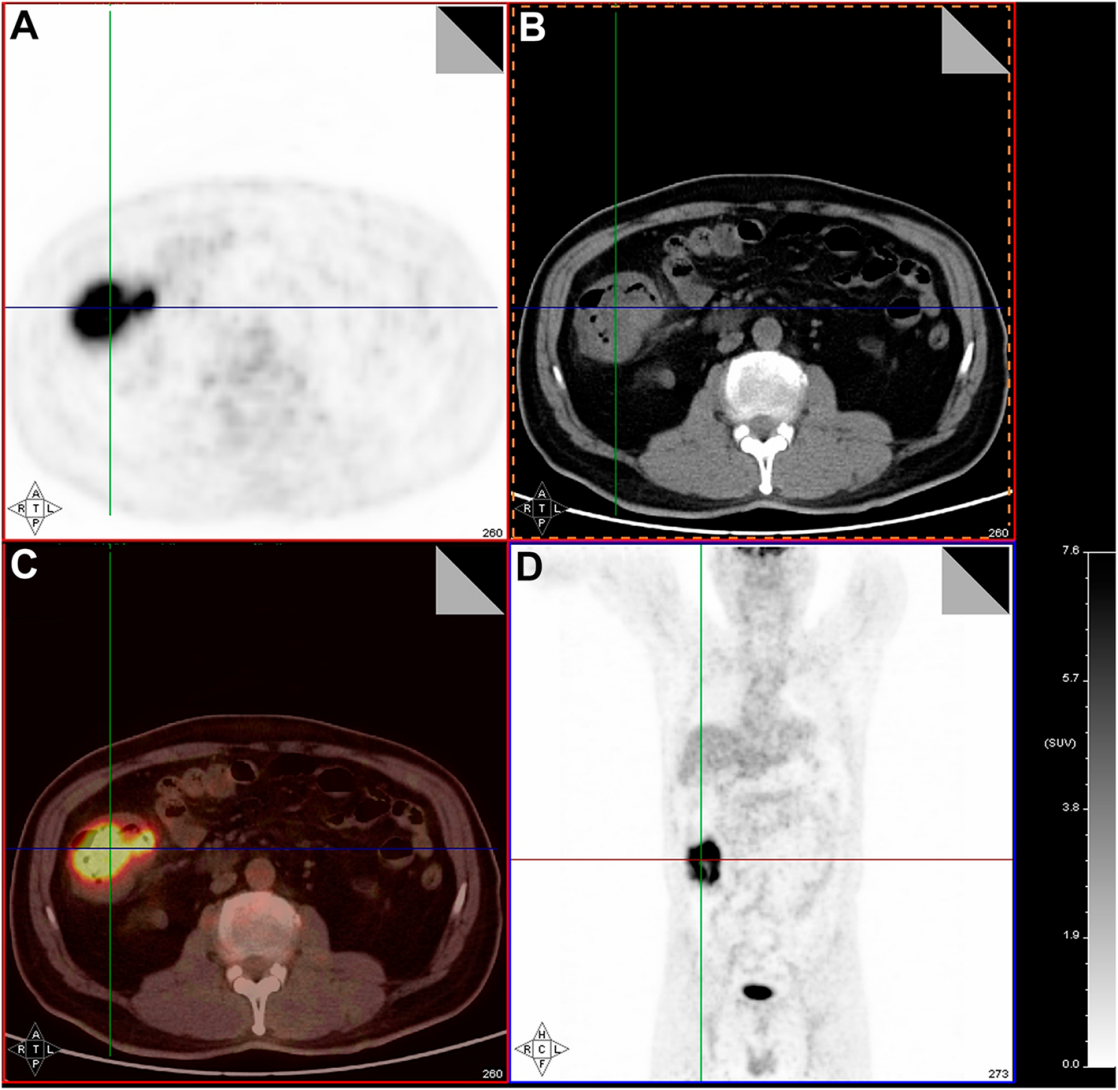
Case study of a patient with ascending colon cancer. a Transaxial PET image showing focal FDG uptake in the ascending colon, taken at the level indicated by the line. b Transaxial CT image of the same lesion. c Image obtained by merger of the PET and CT images. d Coronal PET image of the same lesion
